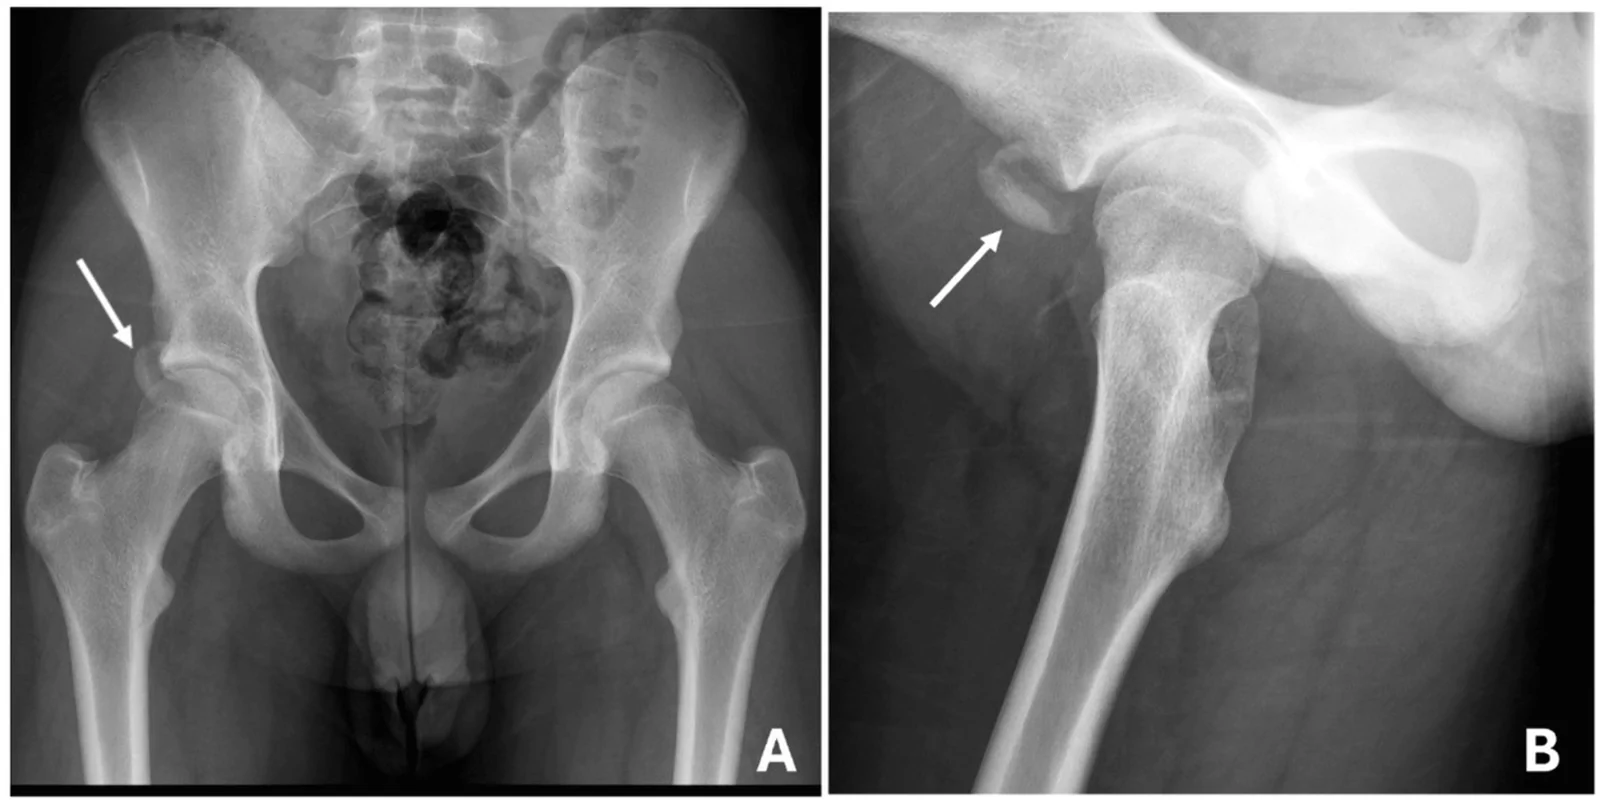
Plain radiographs of the pelvis AP (A) and oblique right hip joint (B) showing well-corticated "L"-shaped bony protuberance from right iliac bone, which extend toward the hip joint, a characteristic of pelvic digit AP: anteroposterior
